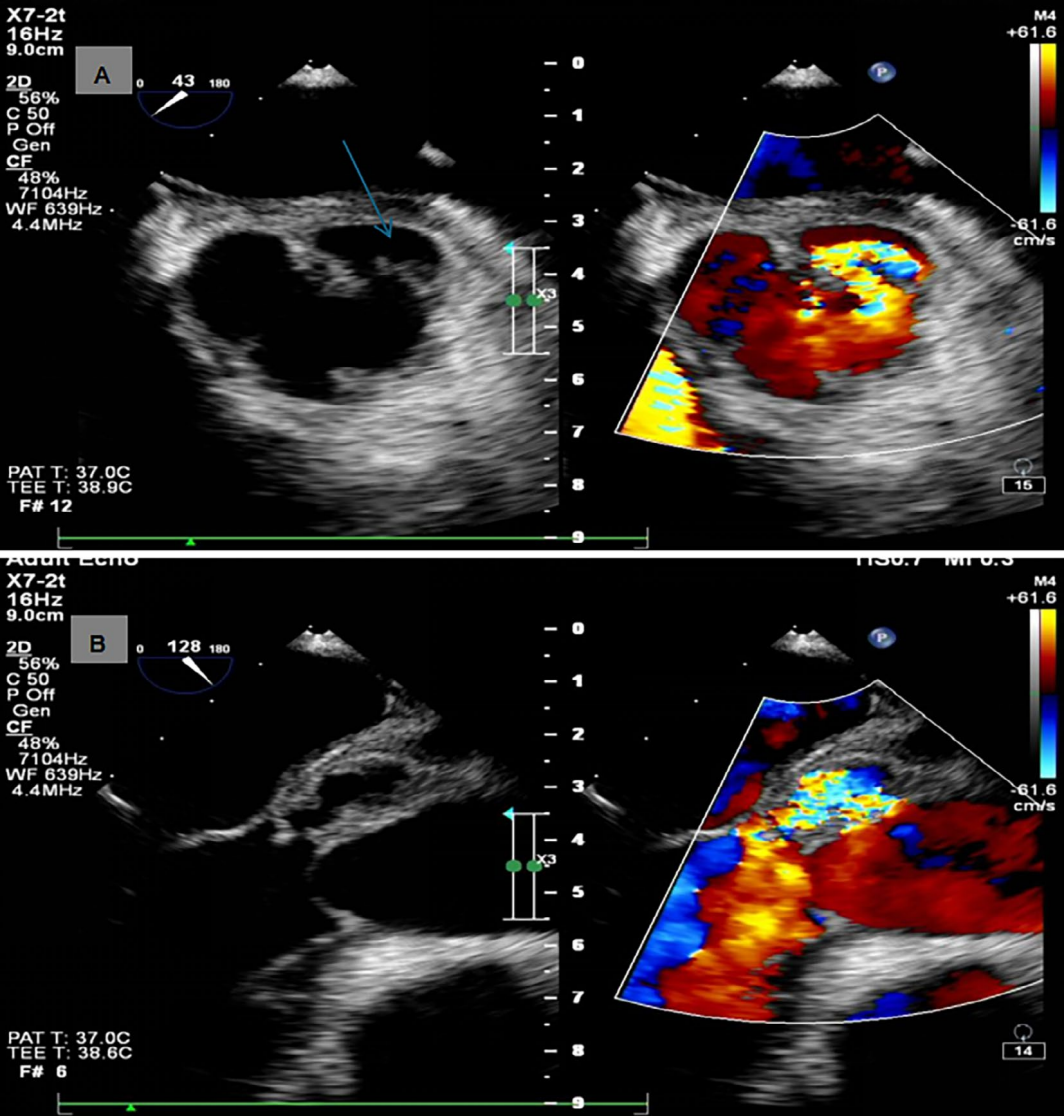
Transesophageal echocardiography imaging. (A) Aortic valve short‐axis with color‐flow Doppler, arrowhead points to periannular abscess. (B) Aortic valve long‐axis with color‐flow Doppler.
